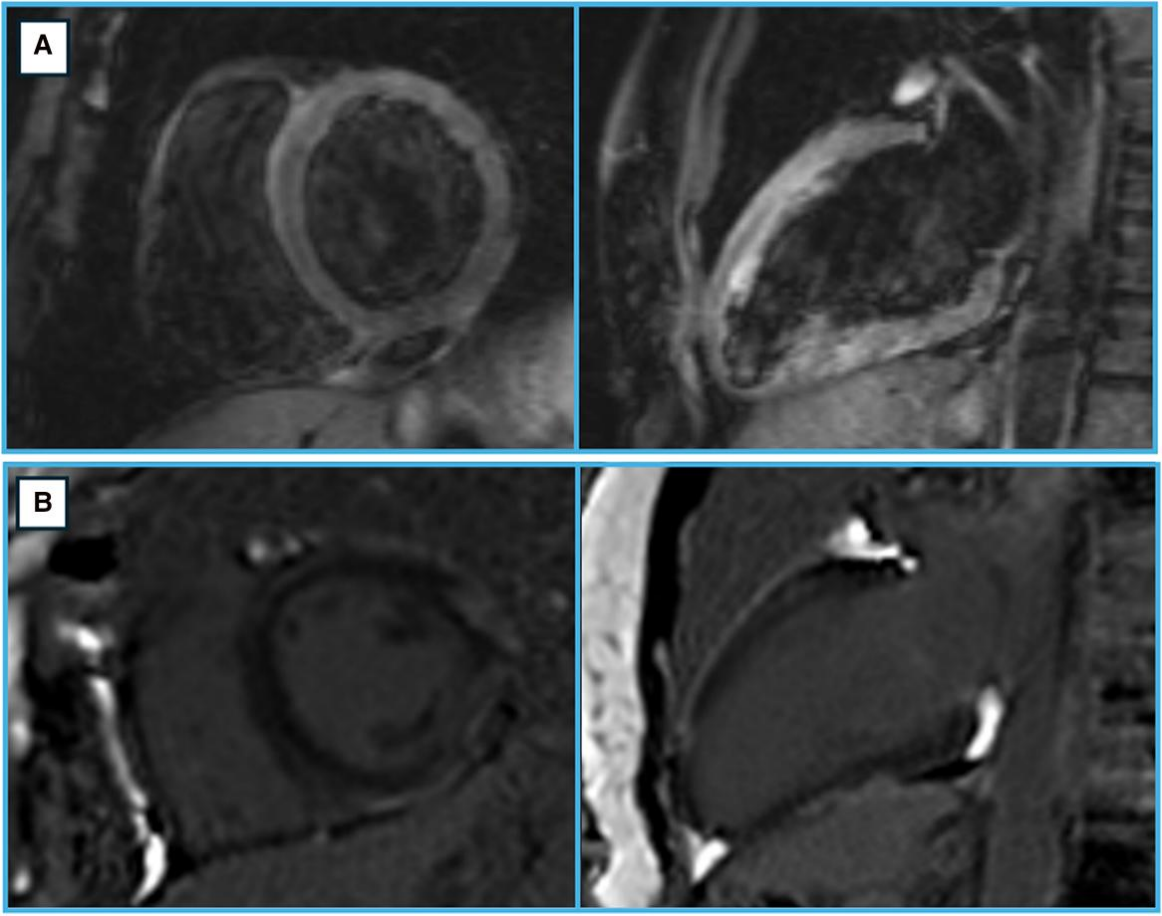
Cardiac magnetic resonance imaging during the acute phase of the first episode (2012). Basal short-axis and vertical long-axis T_2_-weighted sequences show signal hyperintensity in the basal and medial segments of the anterior and anterolateral walls (*A*). In the same segments LGE with a subepicardial and intramyocardial distribution is present, but it can be found also in the proximal and medial segments of the inferolateral and inferior walls. (*B*). These findings are consistent with myocardial oedema. 2018 Lake Louise Criteria for myocarditis are met. CMR, cardiac magnetic resonance; LGE, late gadolinium enhancement
